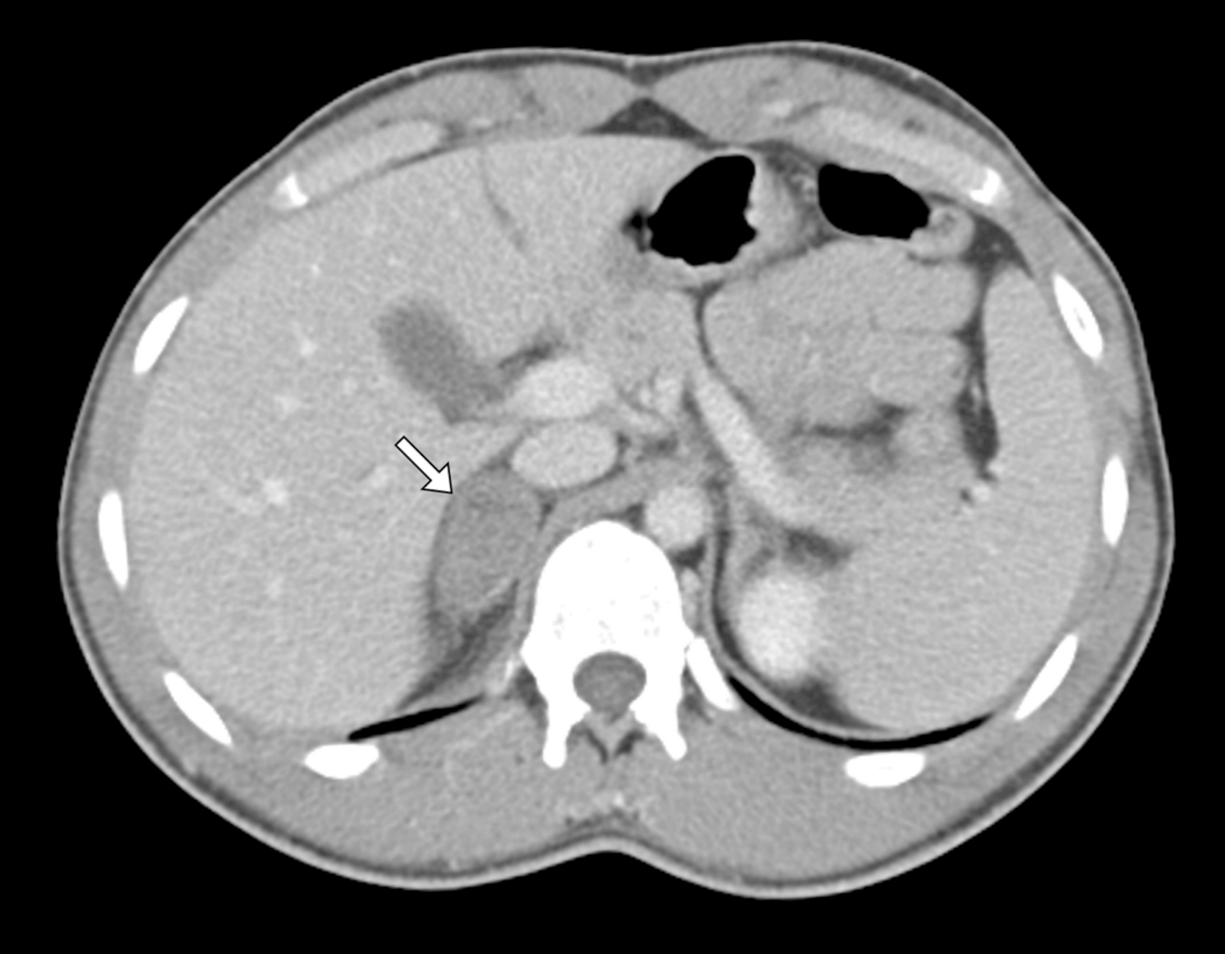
Contrast-enhanced CT demonstrating right adrenal hematoma Contrast-enhanced axial CT image of the abdomen shows a well-defined, non-enhancing lesion within the right adrenal gland (arrow). The absence of contrast enhancement and the lesion’s location are consistent with an adrenal hematoma in the appropriate clinical setting CT: computed tomography
